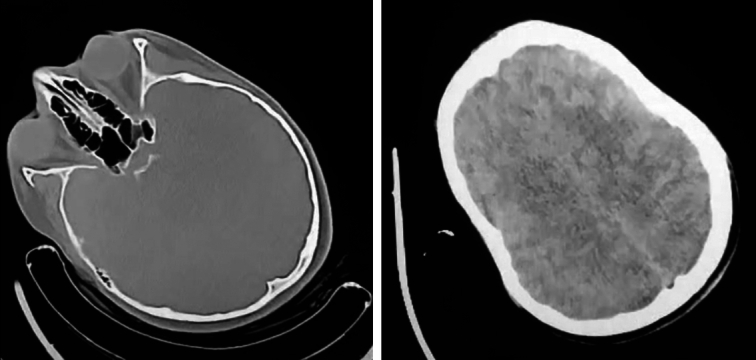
Patient’s brain computed tomography scan revealing normal tissues with no radiological abnormal findings.
